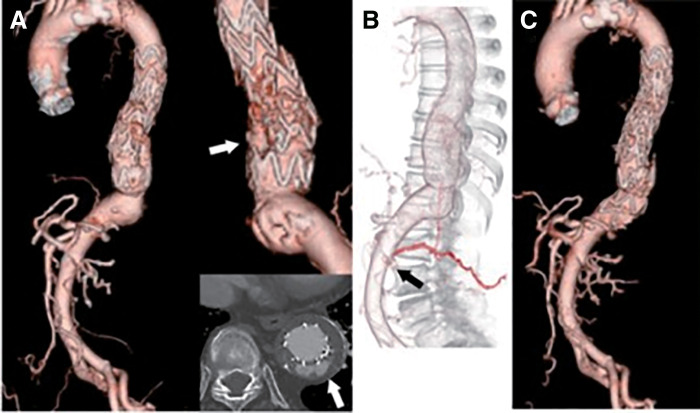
Contrast-enhanced CT findings related to the additional TEVAR. (A) The descending aortic aneurysm is re-enlarged to 54 mm with a type Ib endoleak (white arrow). (B) The ICA-AKA, originating from the left L1 lumbar artery (black arrow), differs from the previously identified ICA-AKA. (C) An additional zone 4 TEVAR was performed to extend the distal landing zone without covering the newly identified ICA-AKA. TEVAR: thoracic endovascular aortic repair; ICA-AKA: intercostal artery branching of the Adamkiewicz artery; CT: computed tomography
